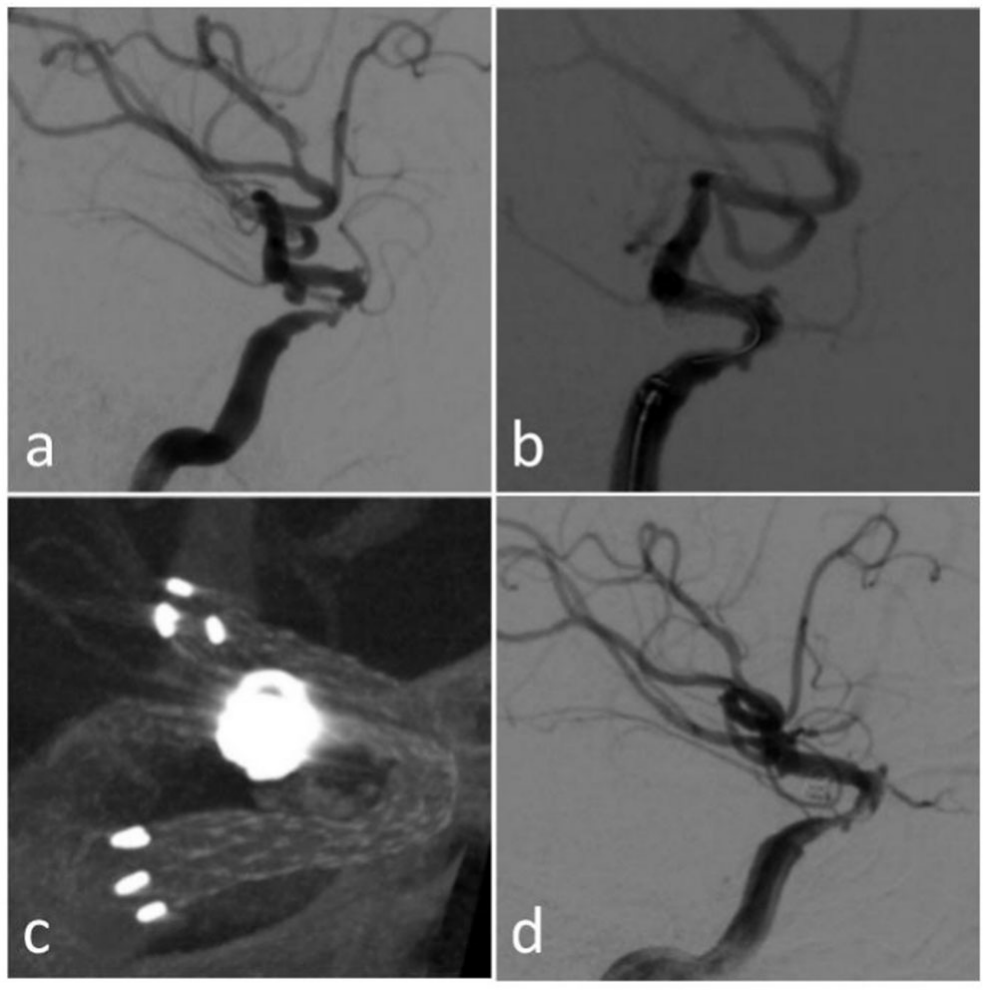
Case 9 (a) original DSA; (b) immediate angiography after the endovascular treatment; (c) Xper CT during the procedure; (d) follow-up angiography after 3 months.
